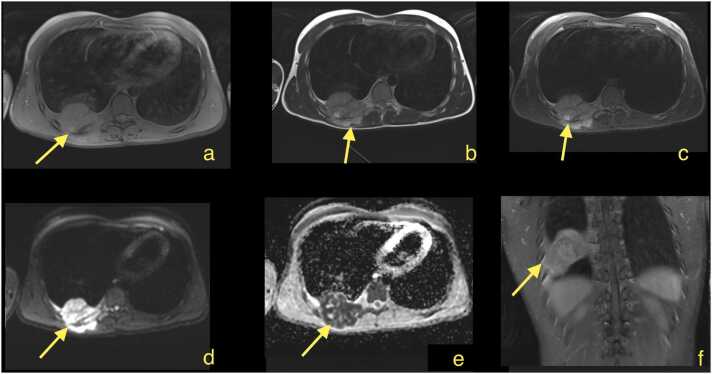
23-year-old patient with Ewing sarcoma. Magnetic resonance imaging showed a solid tumor in the thoracic wall measuring more than 4 cm, with discrete central necrosis.: A - T1-weighted with fat suppression, b - T2-weighted, c - T1-weighted with gadolinium contrast, d - diffusion-weighted imaging (DWI, b 800), e - apparent diffusion coefficient (ADC), f - T1-weighted with gadolinium contrast and with fat suppression.
